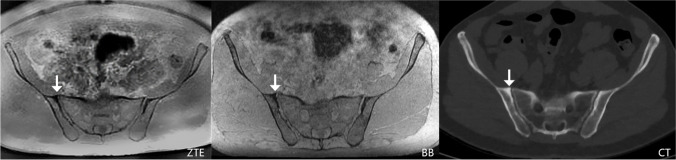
This 62-year-old female patient was referred for liver pathology workup and demonstrated subchondral sclerosis on the right ilium, adjacent to the SI joint (arrow). Note the high contrast between sclerotic and trabecular bone on ZTE and CT as well as reduced “blooming” artifacts of bowel gas on ZTE compared to BB. Abbreviations: ZTE = zero echo time; BB = black bone; CT = computed tomography; SI = sacroiliac joint
